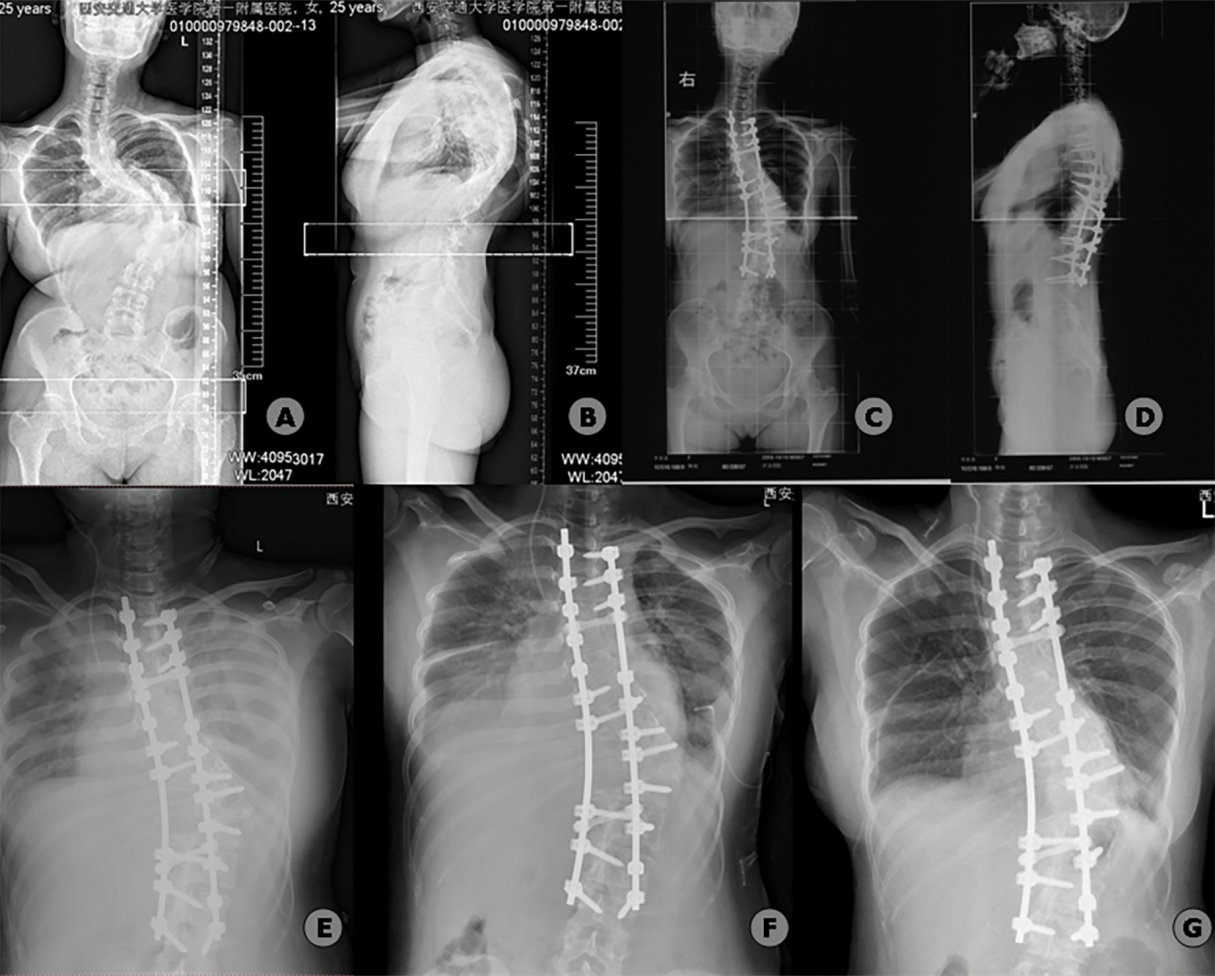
Radiographic findings for a 25-year-old woman with congenital scoliosis who developed postoperative pulmonary complications (bilateral pneumonia and bilateral pleural effusion) after posterior instrumentation, posterior T11 vertebral column resection, and correction and fusion surgery. Preoperative radiographs (A, B) demonstrate a left main thoracic curve of 120°, which was corrected to 38° postoperatively (C, D). The operation time was 450 min, and the volume of intraoperative blood transfusion was 3200 ml. A bedside chest radiograph taken on the third postoperative day (E) and the thoracic ultrasound showed bilateral pneumonia and pleural effusion. After 5 days of treatment with closed thoracic drainage and antibiotics, the patient’s lung condition improved markedly (F). The pulmonary complications had basically recovered by 8 days postoperatively (G).
